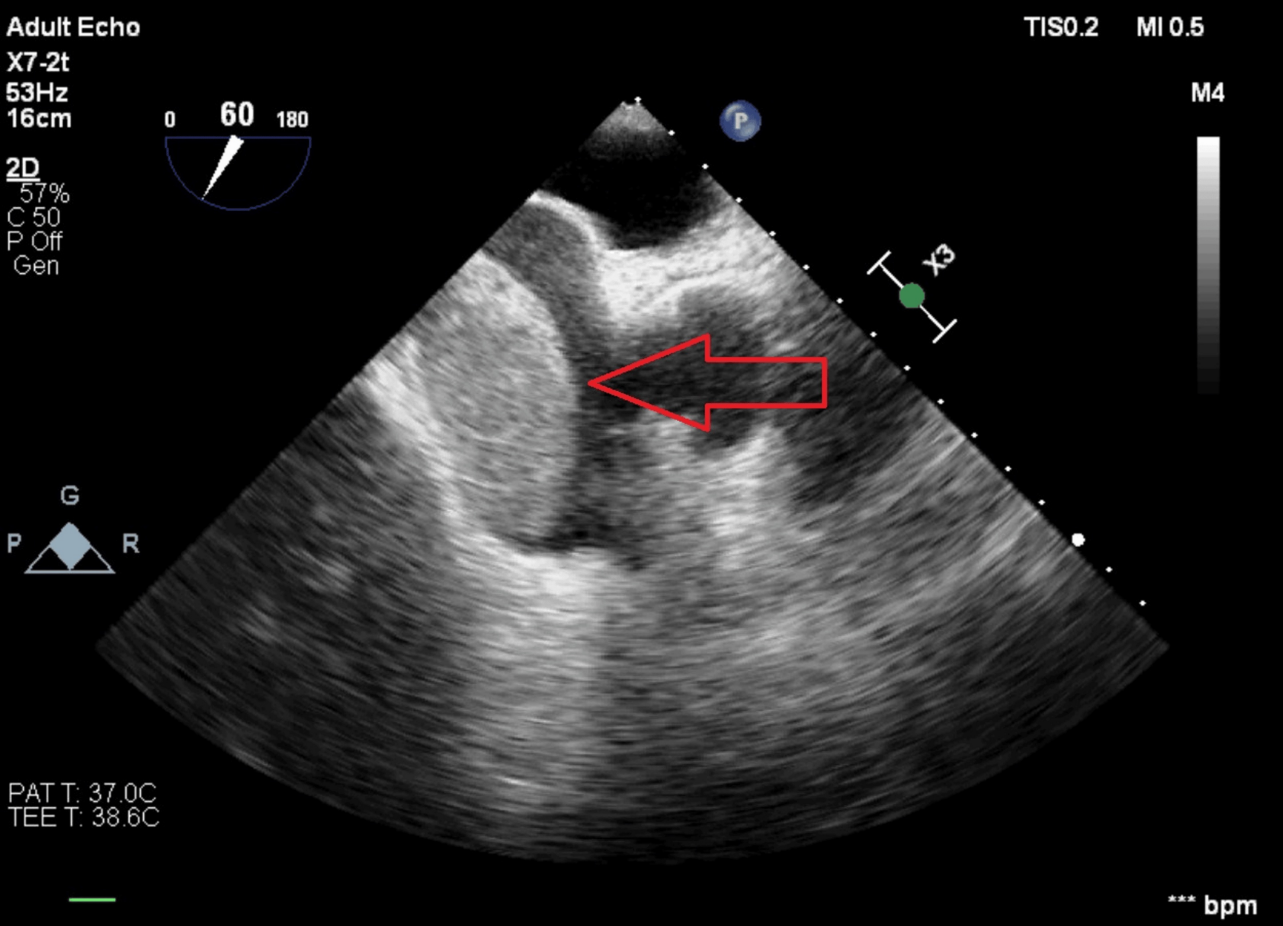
TEE Image Showing the Presence of a Clot in the Right Atria TEE: Trans-esophageal echocardiogram
